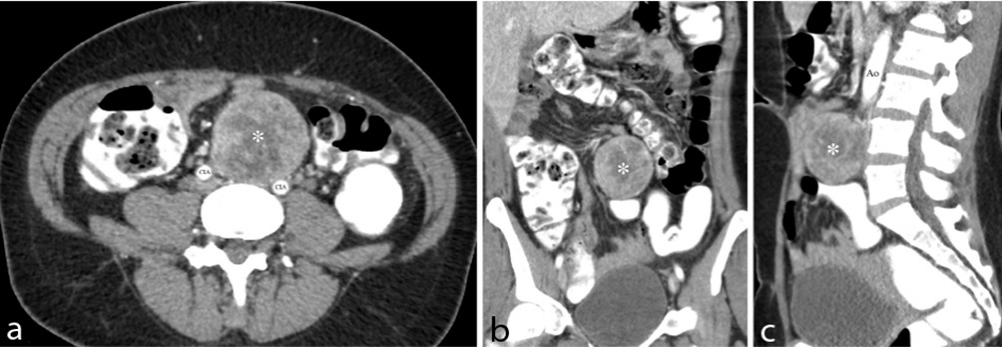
Contrast-enhanced CT with axial (a), coronal (b) and sagittal (c) reconstructions. Heterogeneous enhancing, well-defined soft-tissue mass (asterisk) located retroperitoneal caudal to the aortic bifurcation. There are no signs of an invasive growth pattern. Ao, aorta; CIA, common iliac artery.
